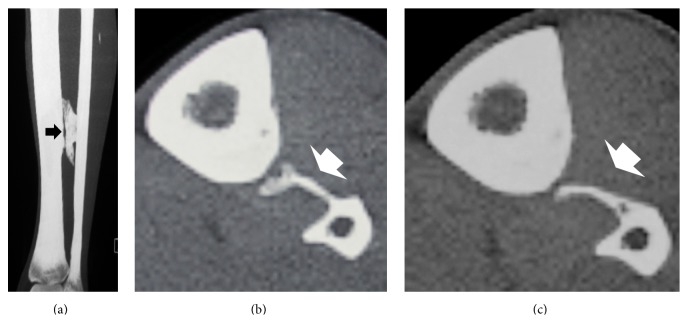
Coronal CT scan of the leg (a) demonstrates that the ossification (*arrow*) is in the interosseous membrane. It is in continuity with the fibula but separated from the tibia by a thin nonossified zone. Axial CT scans (b and c) show separation of the ossification (*arrows*) from lateral border of the tibia.
